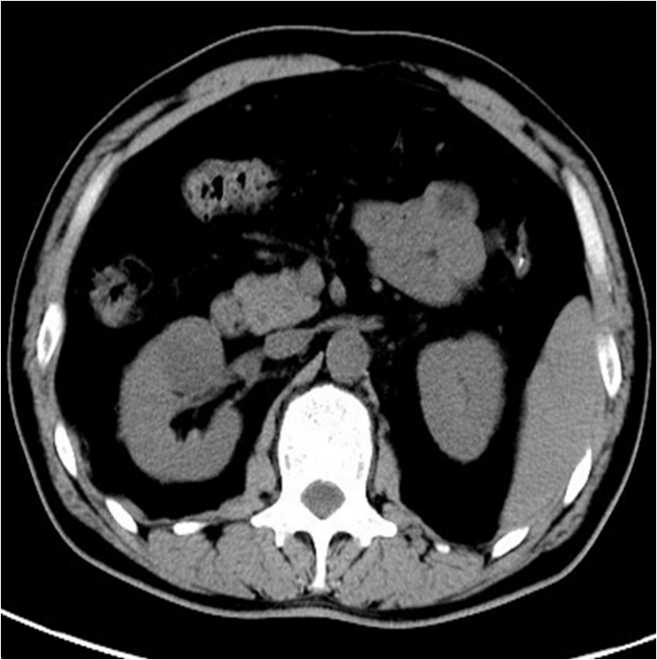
CT showing a heterogeneous or centrally located low-attenuation mass.
